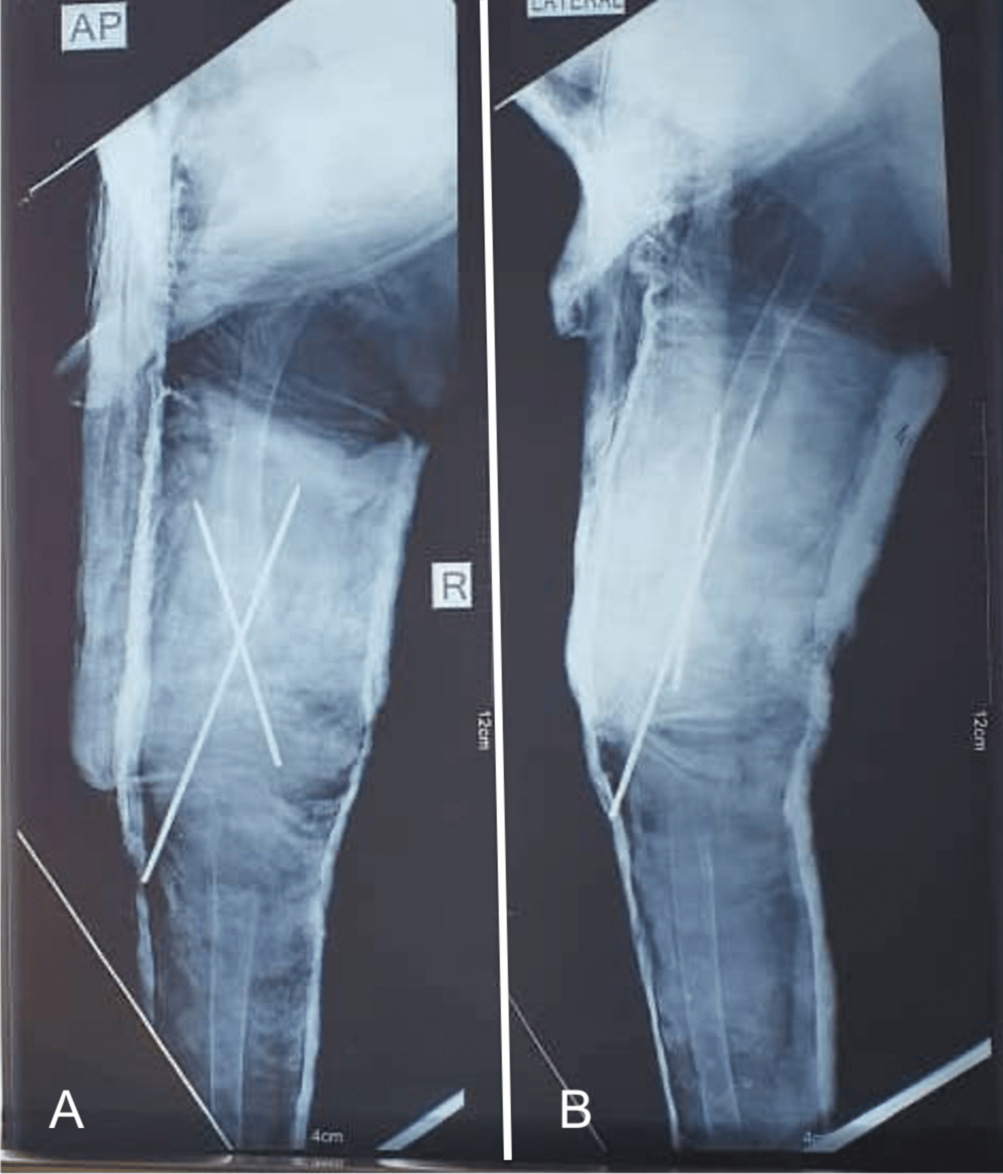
Postoperative X-ray following the second stage of the Masquelet technique. Pins still in place with dangerous advancement on the medial side. (A) AP view; (B) Lateral view.
